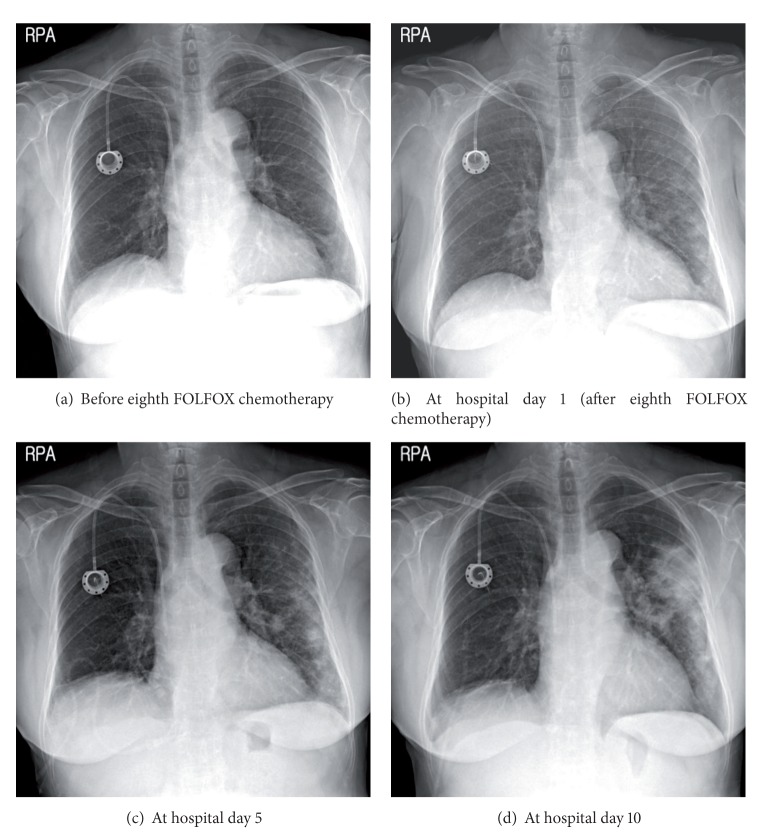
Serial changes in the chest X-ray during admission.
